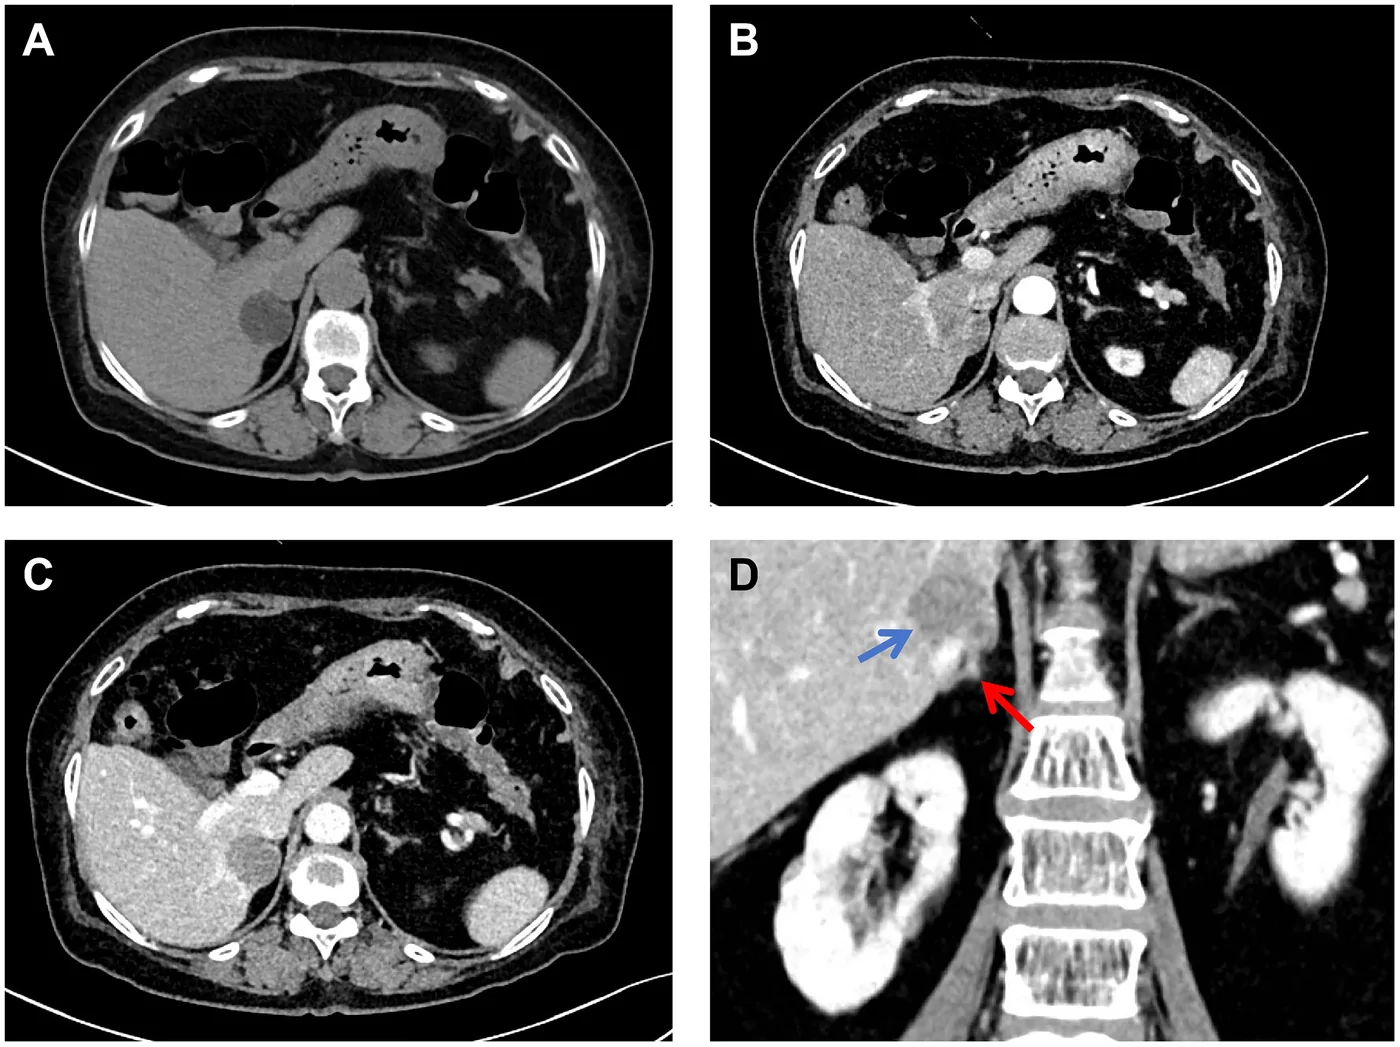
Contrast-enhanced computed tomography findings. (A) Unenhanced CT image showing a well-circumscribed low-attenuation lesion. (B) Arterial phase CT image showing marked enhancement of the lesion. (C) Portal venous phase CT image showing persistent enhancement of the lesion. (D) Coronal reconstructed image demonstrating the anatomical relationship between the tumor (blue arrow) and the right adrenal gland (red arrow).
